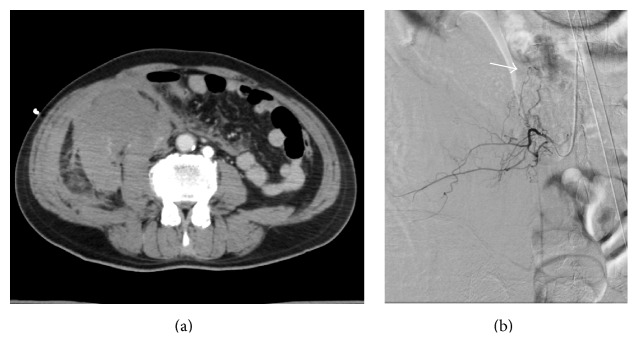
(a) Computed tomography of the abdomen revealed a large hematoma in the right abdomen. (b) Selective angiography revealed bleeding (arrow) from the right lumbar artery.
